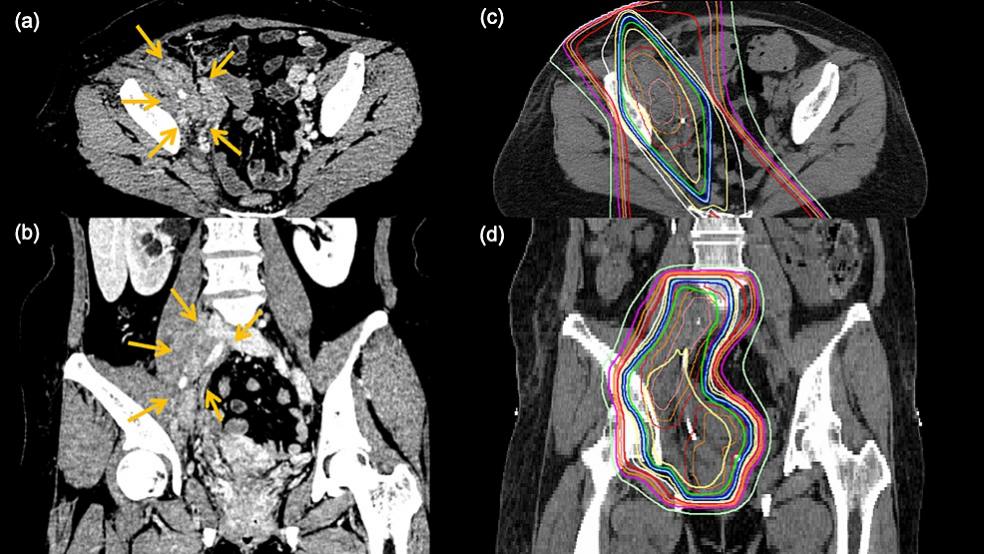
a 54-year-old woman with stage IV uterine cervix cancer (patient 5) (a) and (b): axial and coronal views of the tumor (arrows) on CT before irradiation. The numerous enlarged lymph nodes in the pelvis are infiltrating the right iliopsoas muscle (allows). (c) and (d): radiation dose distribution with 95% isodose lines shown in green.
